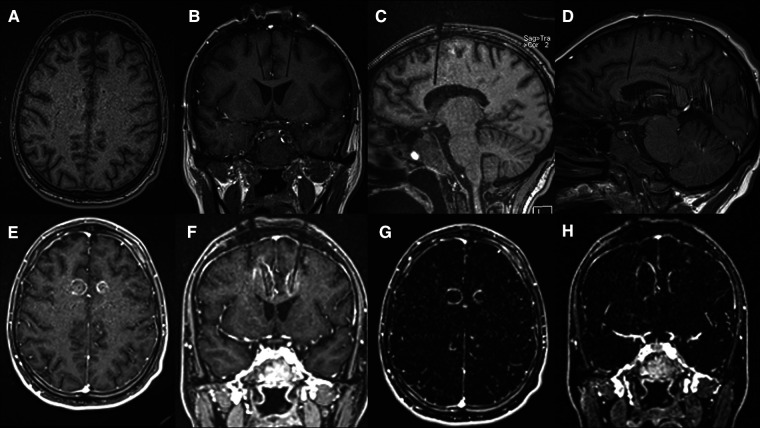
(A–D) Pre-ablation T1 sequences obtained for confirmation of the laser catheter location immediately before ablation. Axial (A), Coronal (B) and Sagittal (C – Left and D – Right); (E–H) Post-ablation T1 + C axial (E) and coronal (F) images and MRI subtraction, [i.e. (T1 + C)-T1] axial (G) and coronal (H) slices (G,H) demonstrating the area of tissue damage highlighted by surrounding ring enhancement.
